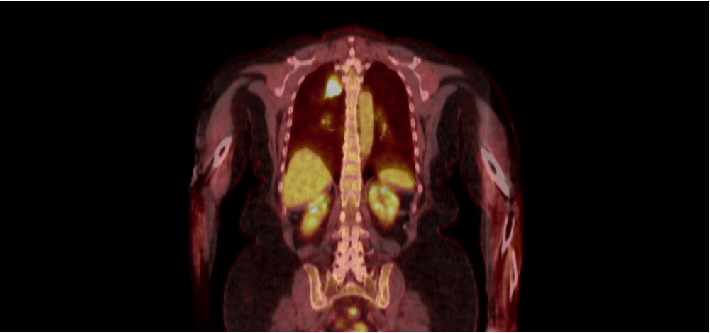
PET CT showing a hypermetabolic right upper lobe nodule.
